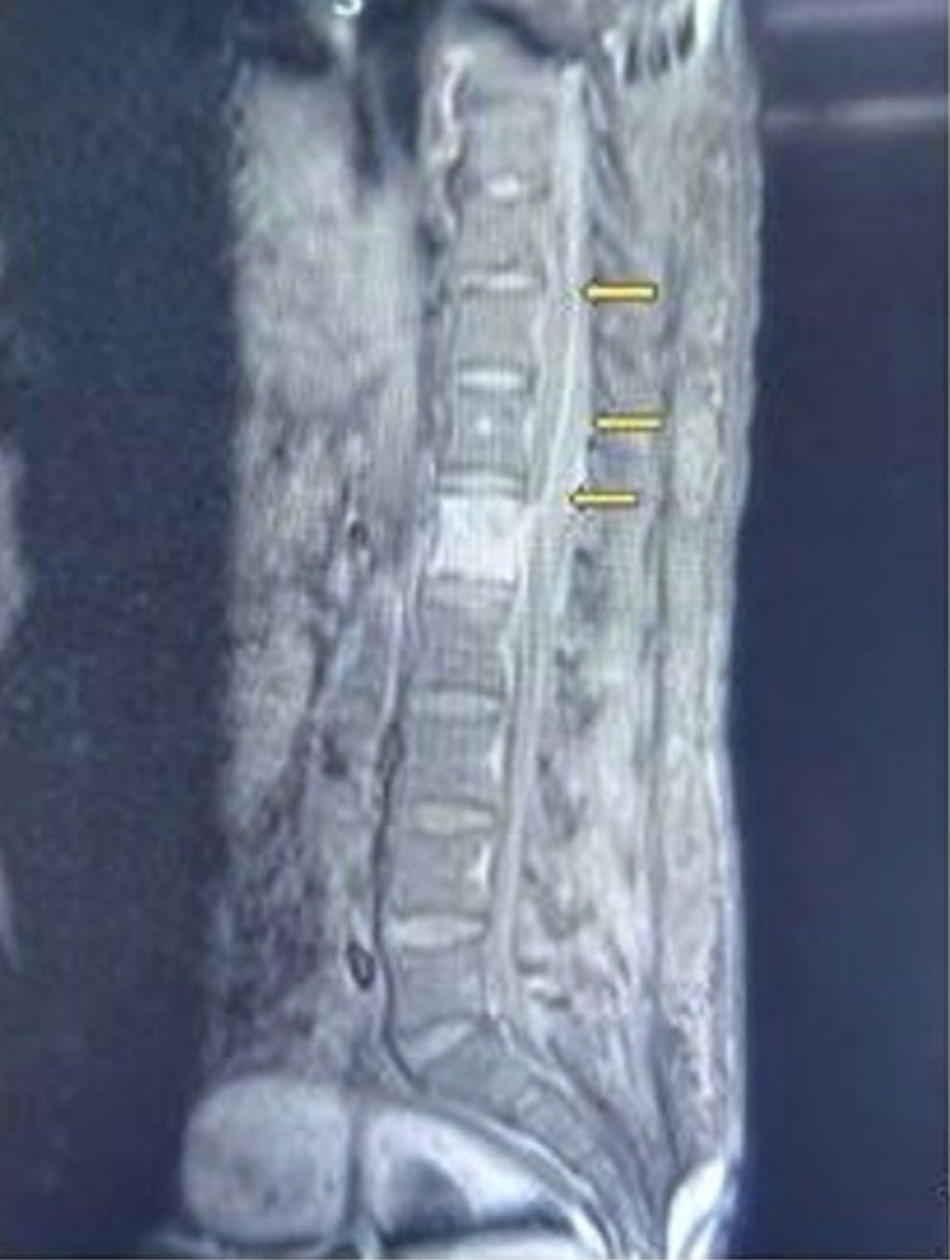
An MRI of the dorsolumbar spine showed an elongated area of peripherally enhancing altered signal intensity lesion in epidural space extending from D9 to L2 levels.
